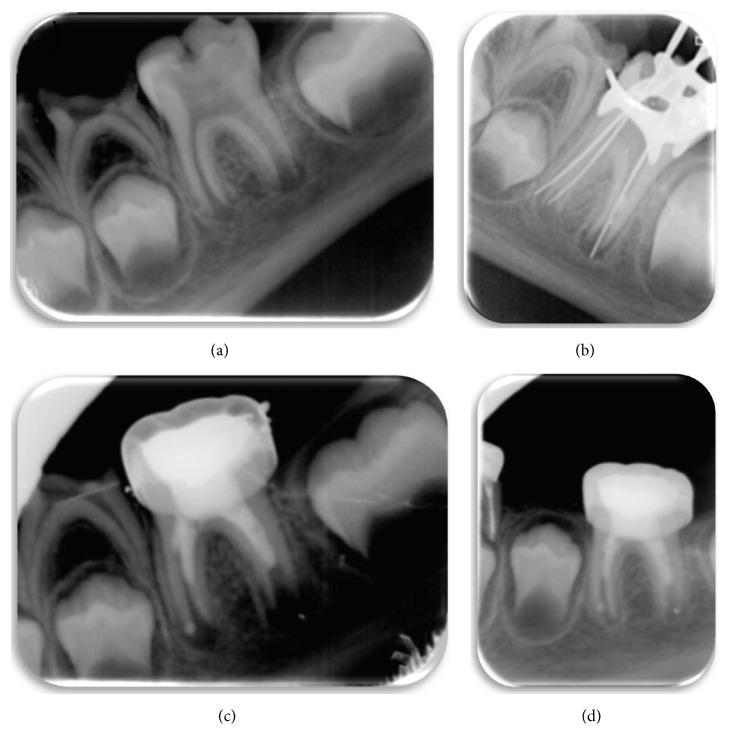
(a) Initial periapical radiograph: occlusal caries can be observed extending into the pulp chamber and radiotranslucency on the root apices. (b) A size 15 K-file is used to irritate the apical tissue and stimulate bleeding. (c) Completion of revascularization. (d) Follow-up periapical radiograph, 8 months after completion of revascularization. The periapical radiotranslucency has disappeared and the walls of the root canal have thickened; apical closure has still not taken place. This should occur at the following check-up.
